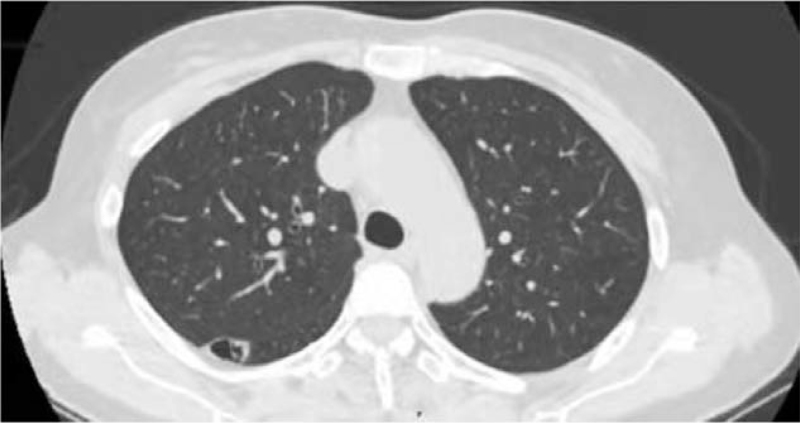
Chest computed tomography scan: the nodule with a thin wall bulla was located in the dorsal segment of the right lower lobe, with a size of 2.0 × 0.8 cm.
